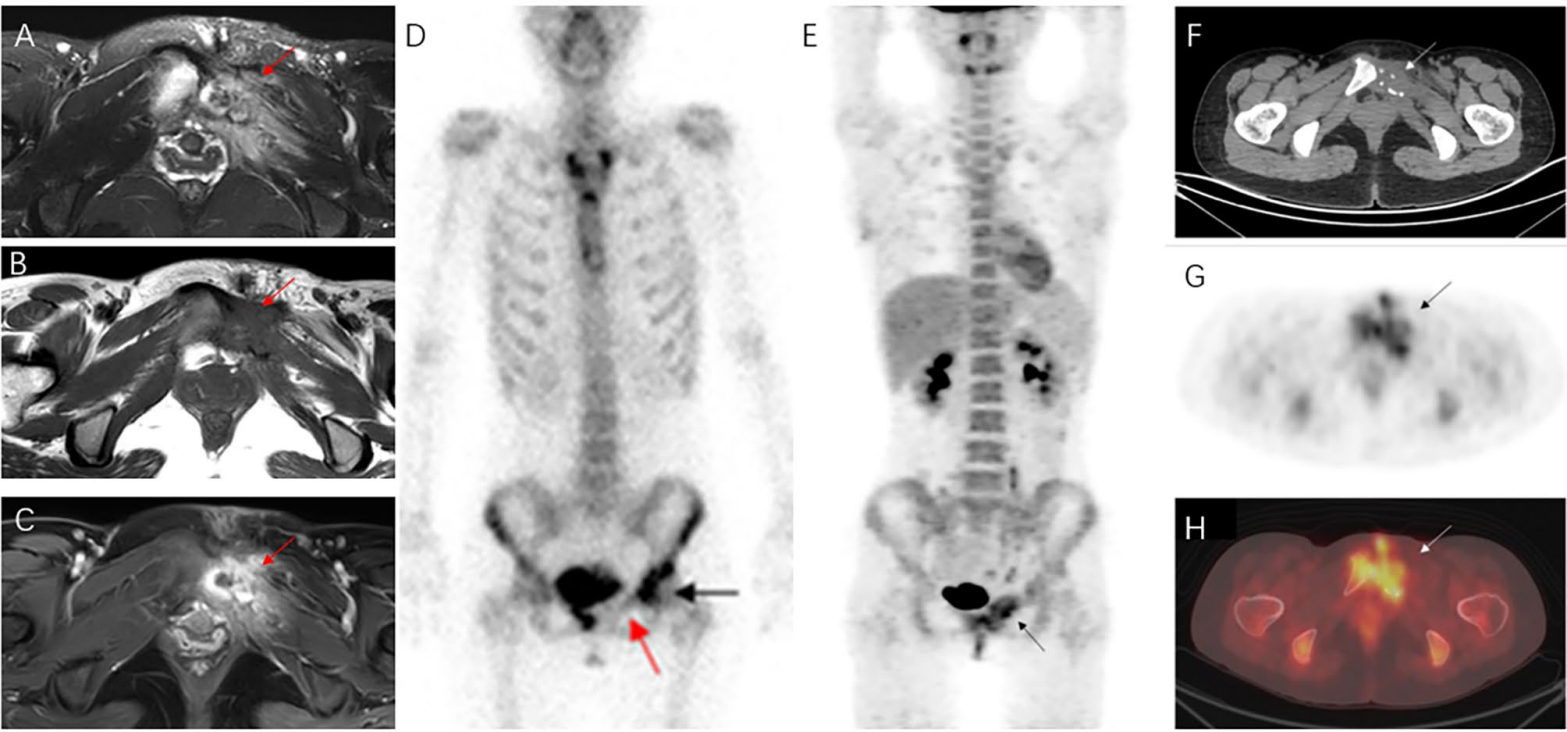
The lesion showed high signal intensity on the fat-suppressed sequences of T2-weighted MR images (A, arrow) and isointense signal intensity on T1-weighted MR images (B, arrow), with a size of about 36 mm×35 mm. After injection of the contrast agent (Gd-DTPA), the lesion showed inhomogeneous enhancement (C, arrow). Bone marrow edema of the right pubis was shown on MRI. A 99mTc MDP bone scan showed that this lesion was radioactively absent (D, red arrow), while the adjacent left acetabulum was radioactively concentrated because of reactive osteosclerosis (D, black arrow). The maximum intensity projection (MIP) PET (E), transverse CT (F), corresponding PET (G), and fusion (H) images showed increased FDG uptake of the osteolytic lesion (arrows) with SUVmax of 5.0. Except for this lesion, no abnormal FDG uptake was observed in any other parts of the body
